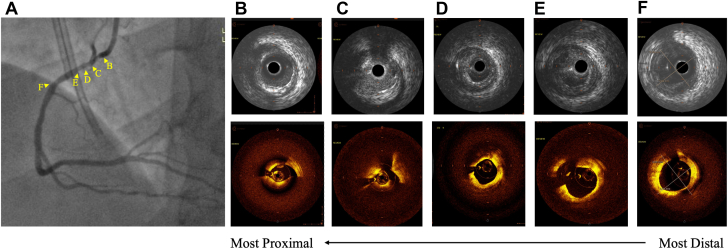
Before percutaneous coronary intervention. (A) Angiogram of right coronary artery (RCA) lesion; intravenous ultrasound (top) and optical coherence tomography (bottom) visualizations of (B) ostial RCA, (C) conus branch, (D) intraplaque hemorrhage and wire artifact, (E) plaque rupture cavity and wire artifact, and (F) distal reference.
